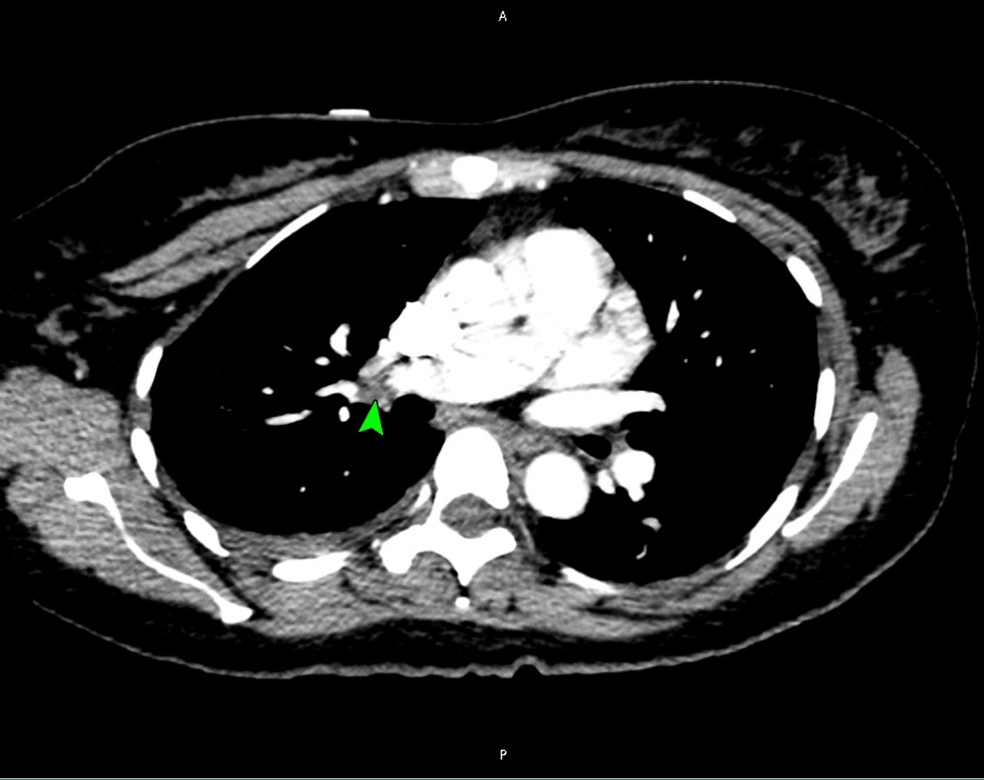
A CT pulmonary angiogram image showing a large pulmonary embolus in the right pulmonary artery (arrowhead) CT: computed tomography
